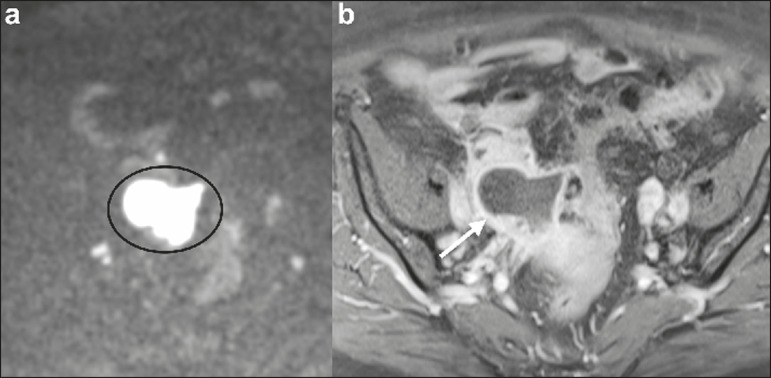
MRI of a female patient with tubo-ovarian abscess. a: Axial
